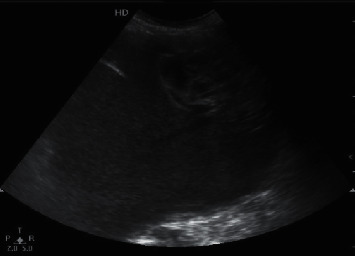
Ultrasound image of ovarian cyst of a female patient who underwent GLS (38 years old).
